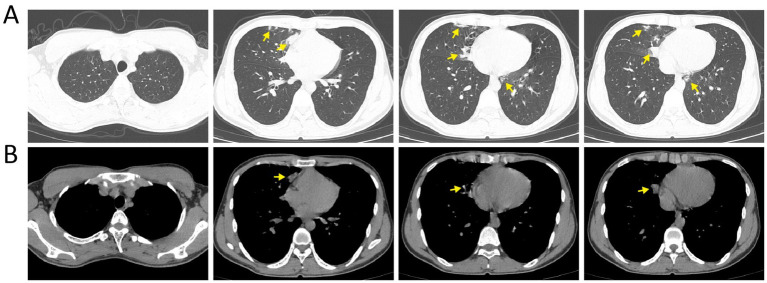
Chest CT on the first day of admission. (A) Lung window; (B) mediastinal window. Yellow arrows indicate the lesion sites.
